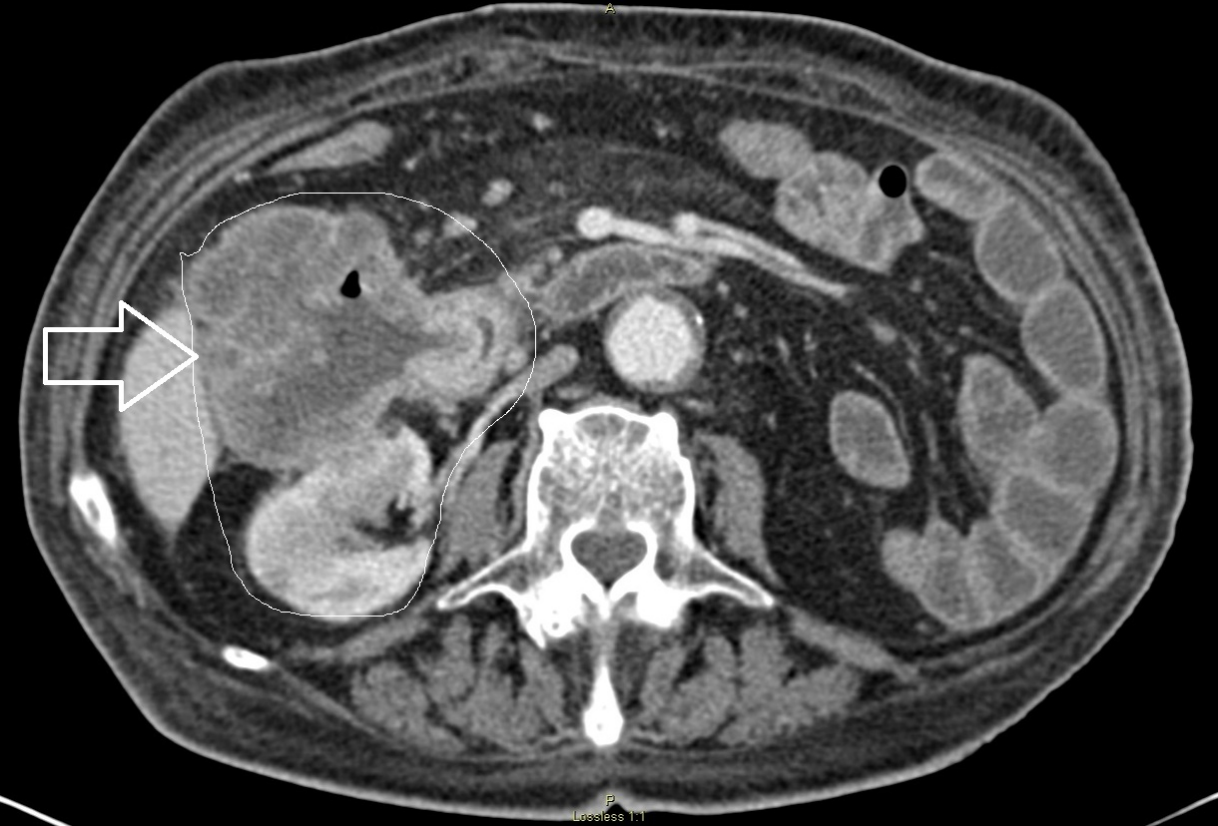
Computed tomography (CT) scan of abdomen/pelvis, axial view showing a right colon (hepatic flexure) mass invading the second portion of the duodenum and the right kidney. Areas of interest outlined, and arrow pointing at the hepatic flexure mass.
